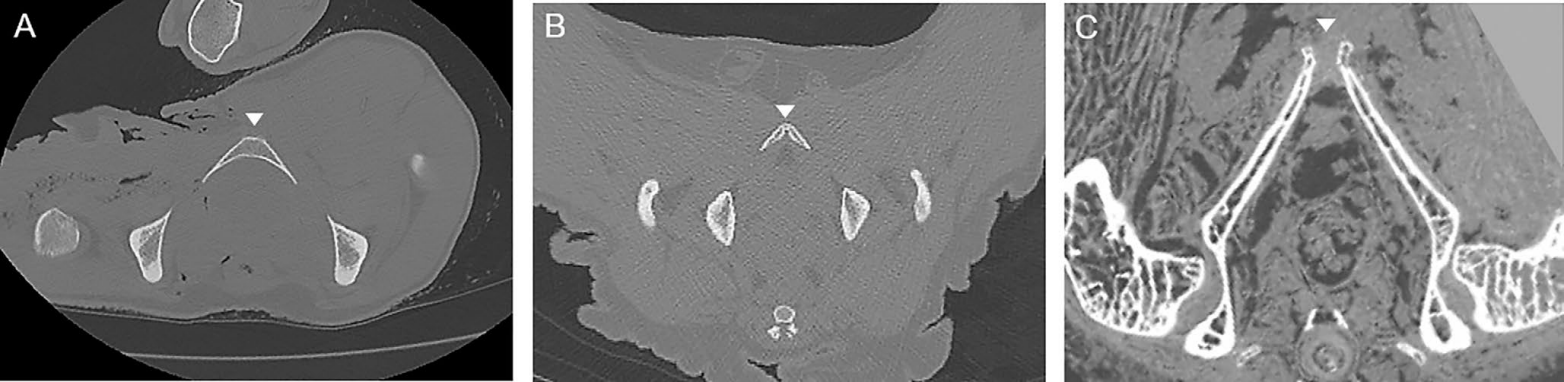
Examination of pubic symphysis condition on CT scan slices, representing a transverse section of the body lying in supine position. The pubic symphysis is indicated by a small triangle. (A) Fully fused pubic bones showing bone remodeling in male *Pa. troglodytes* PRICT‐660; (B) pubic bones showing early stage of fusion in male *Ma. mulatta* UCD‐33734; (C) pubic bones separated by a cartilaginous symphysis in female *Ga. moholi* dlc‐2016f.
